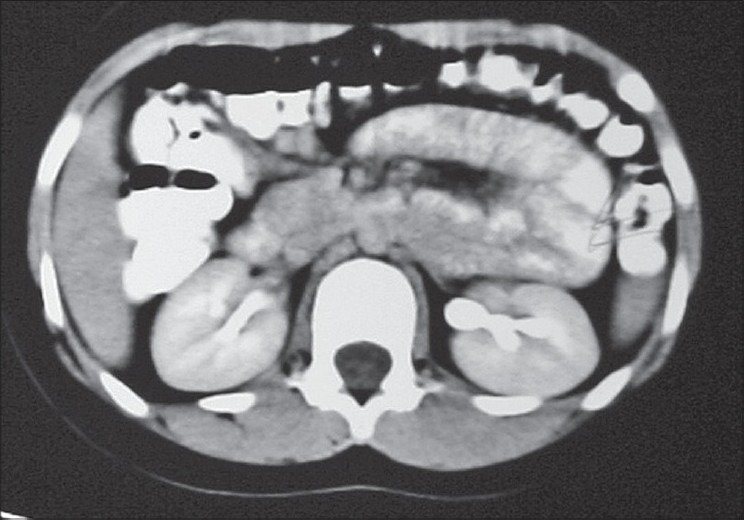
Axial CT, showing distended loop on the left side
